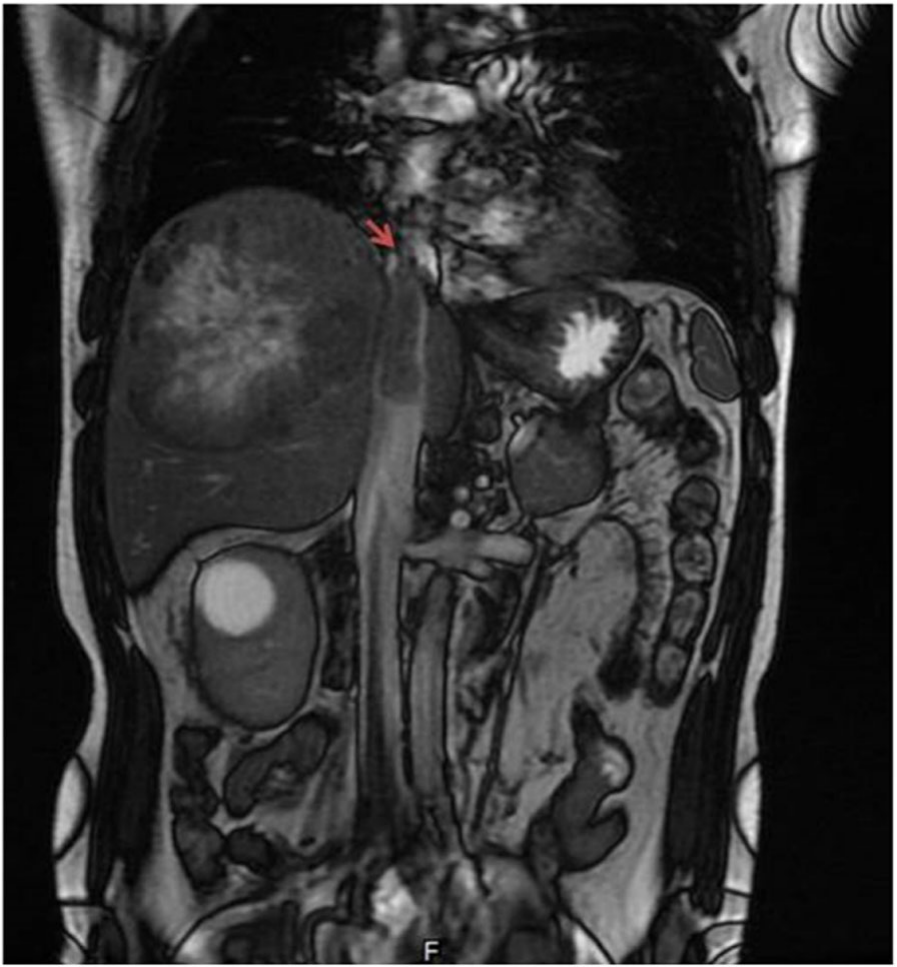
Magnetic resonance imaging of a patient with hepatocellular carcinoma. The *arrow* indicates the thrombi reaching the hepatocaval junction
